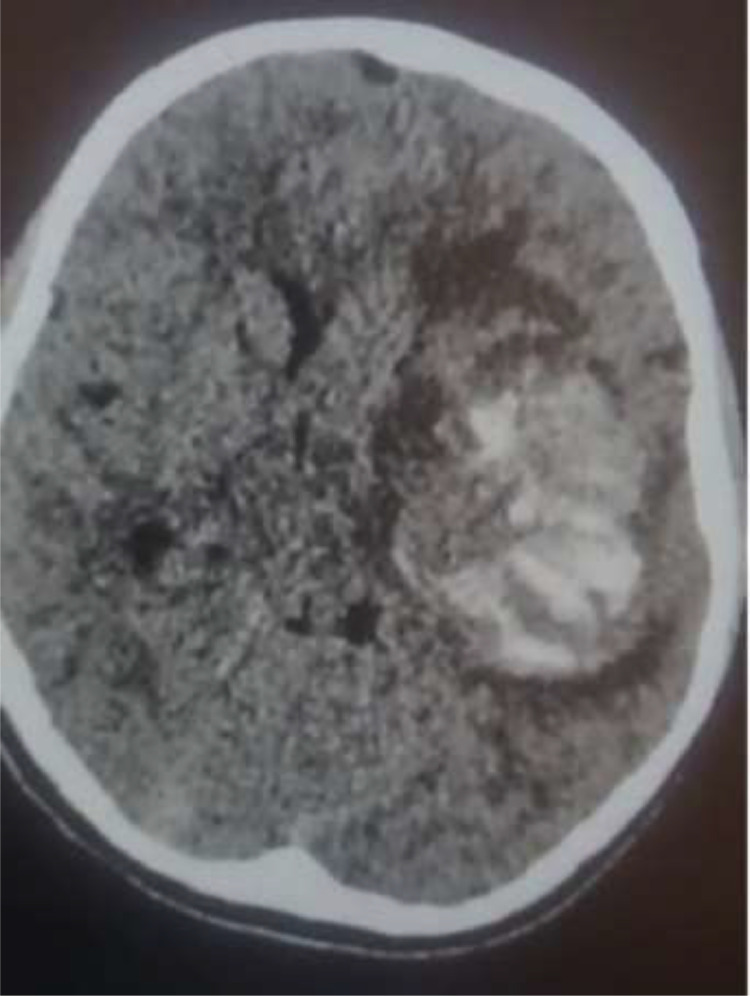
The initial CT brain imaging performed during the patient's second presentation to the emergency department. Non-enhanced CT of the brain shows a large hyperdense mass-occupying lesion in the left frontotemporal region, causing a mass effect on the left lateral ventricle. Also noted is perilesional edema with effacement of the sulci.
